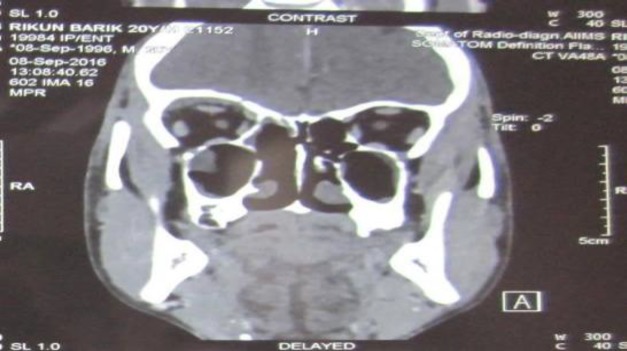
Coronal scan (CT) showing complete clearance of the disease
